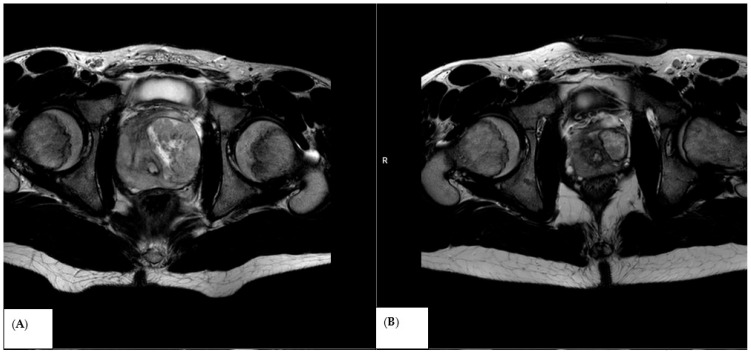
T2-weighted image in the transverse plane of the RMS prostate tumor, (A) before treatment and (B) after 3 cycles of chemotherapy.
